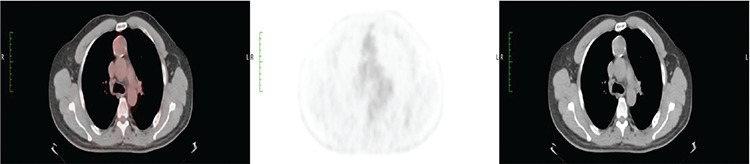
The ^18^F-FDG PET/CT images of a 56-year-old man with anterior mediastinal mass in the transaxial projection of fusion, PET, and CT with low ^18^F-FDG uptake who was out of the follow-up program without any pathological outcome ^18^F-FDG: ^18^F-fluorodeoxyglucose, PET/CT: Positron emission tomography/computed tomography
